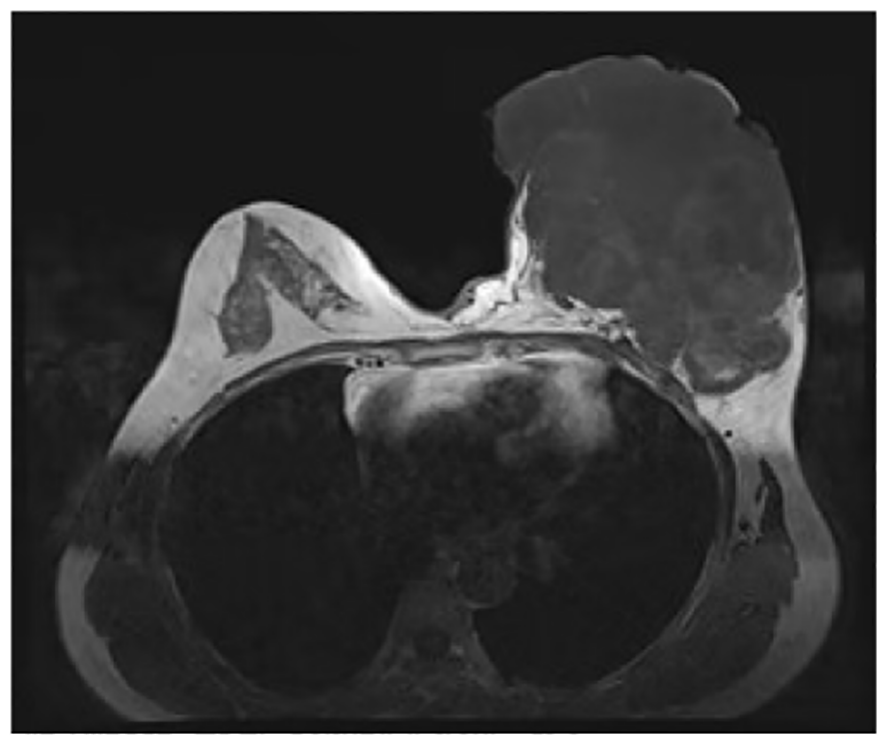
Contrast-enhanced magnetic resonance imaging findings. The boundary between the mass and the pectoralis major muscle was indistinct, with persistent signs of infection observed.
